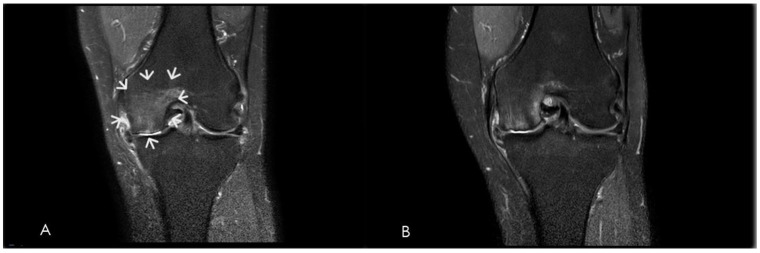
Magnetic resonance imaging (MRI) of the left knee in the same patient of Figure 3. (A) Pre-procedural fat-suppressed T2-weighted sequence showing synovitis and bone marrow edema (arrows) in the medial compartment. (B) One-month post-GAE follow-up MRI demonstrating a marked reduction in synovial hyperintensity and bone marrow edema.
